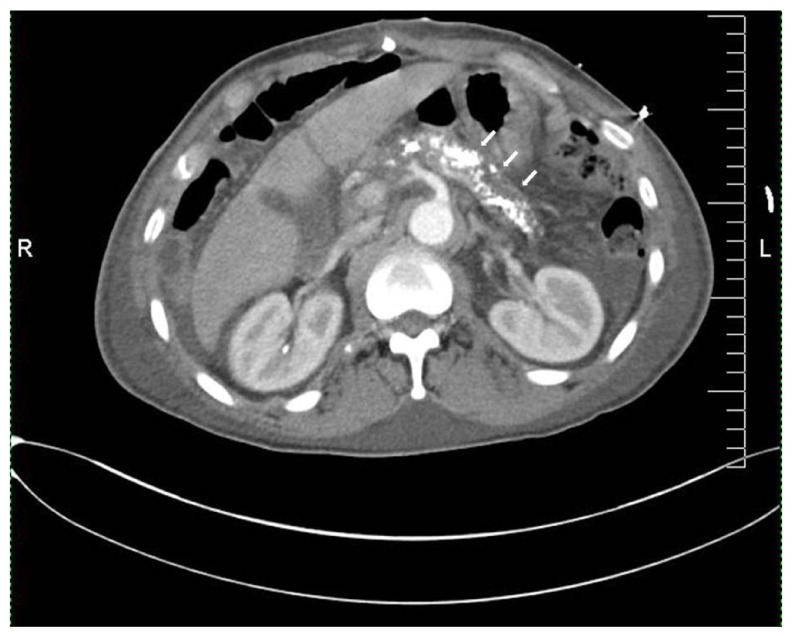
CT Abdomen. Calcifications within the pancreas are shown by arrows.
